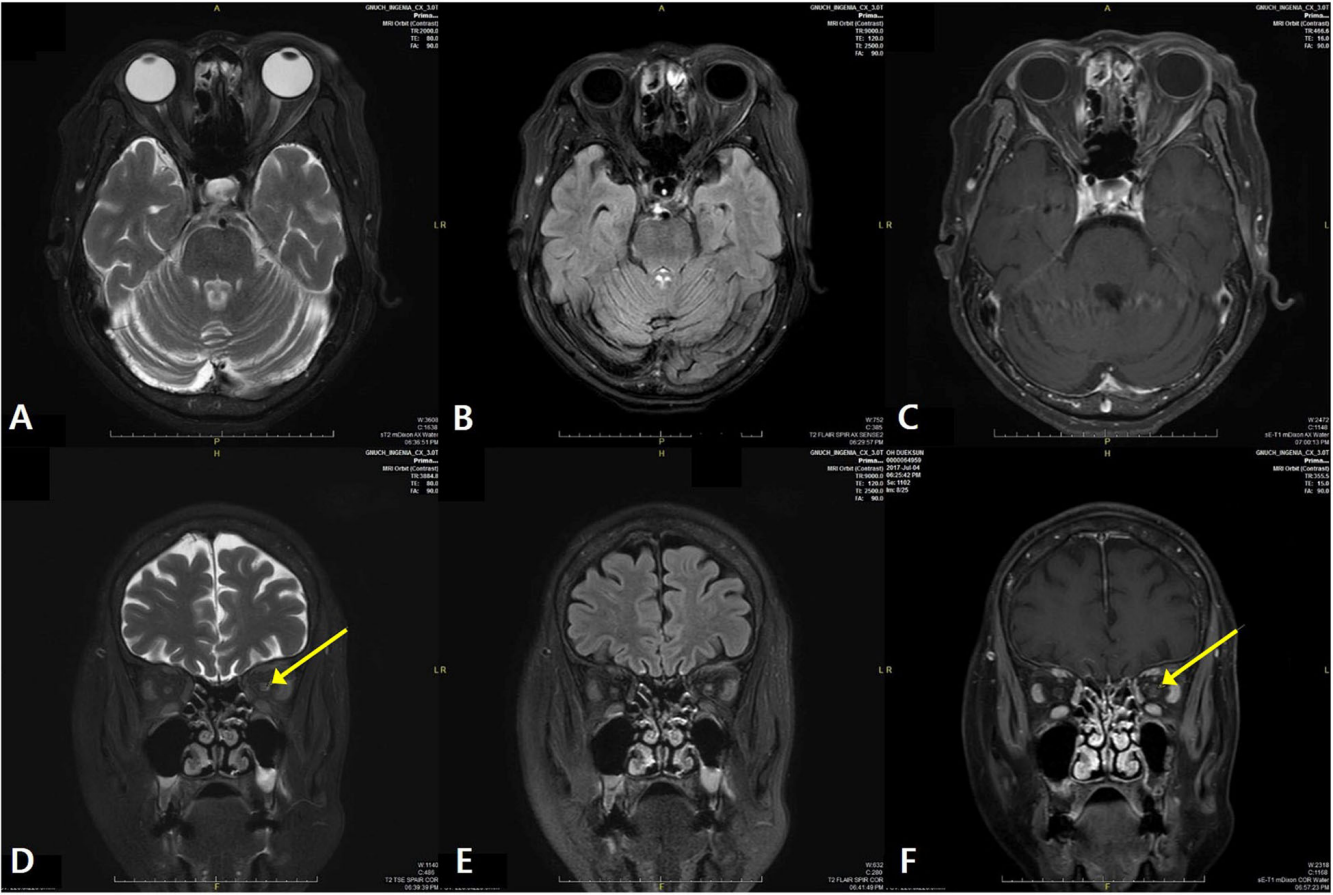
Axial images of brain MRI (a, b and c: T2-weighted image (T2-WI), Flair images, and T1 post contrast images, respectively). Coronal images of brain MRI (d, e and f: T2-WI, Flair images, and T1 post contrast images, respectively). T2-WI (d) revealing hyperintensity in the intra-orbital segment of the left optic nerve (arrow). T1 post contrast images (f) revealing enhancement in the same level (arrow)
